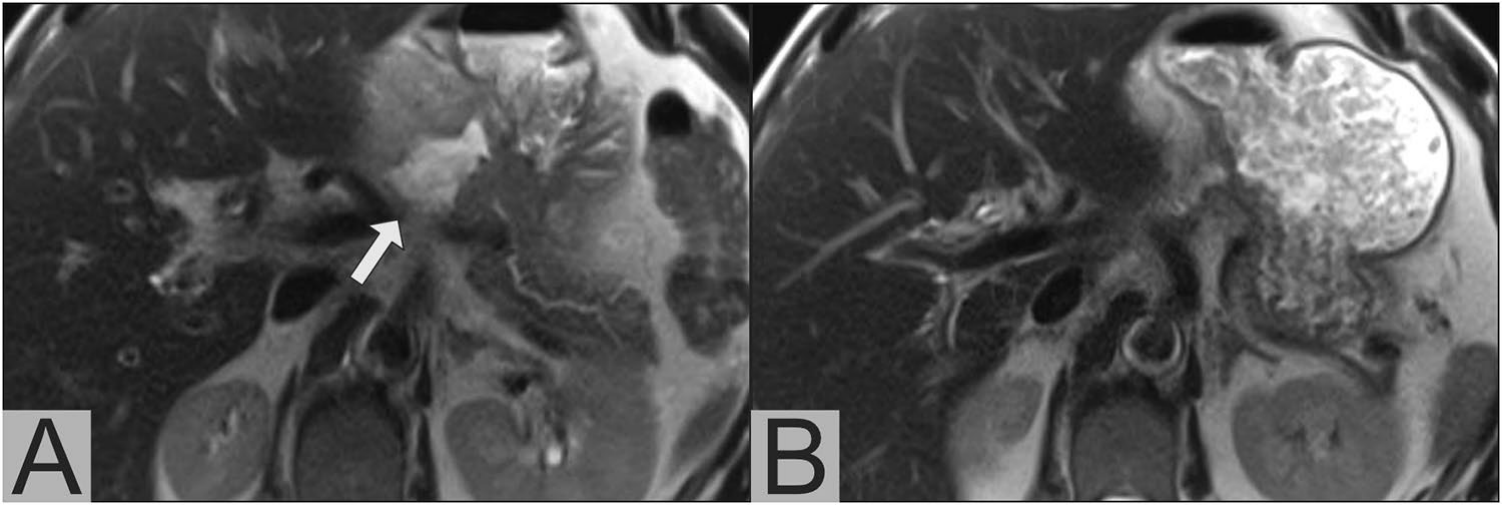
PoSSe MRI shows a small fluid collection next to the pancreaticogastrostomy (a, arrow). After consultation with the surgeon in charge no drain was placed in this collection. In the follow-up PoSSe MRI 5 days later (b), the collection has decreased in size and is barely visible
